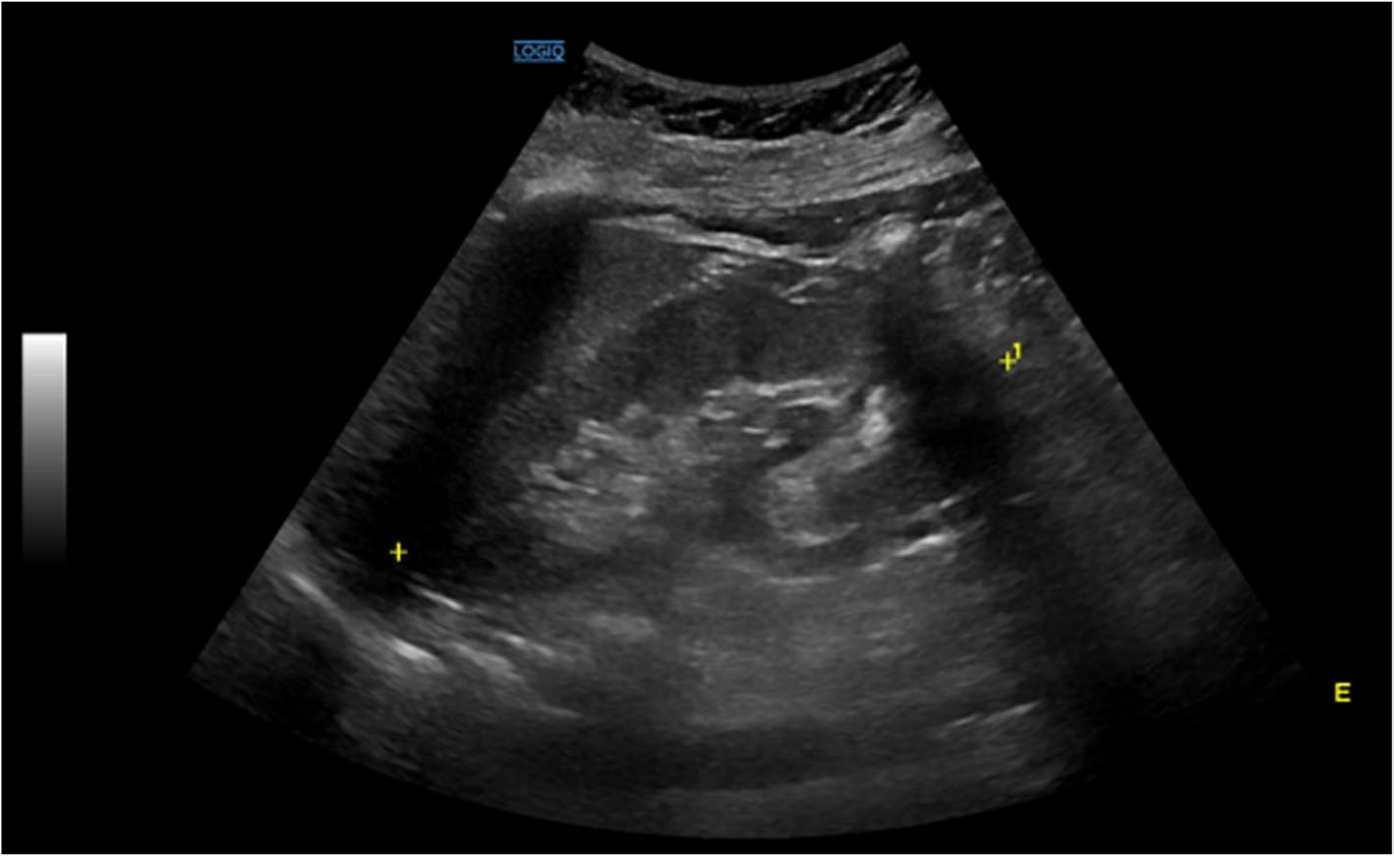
Ultrasonography showing an unspecific 31x18mm solid nodule at the left supraclavicular fossae
